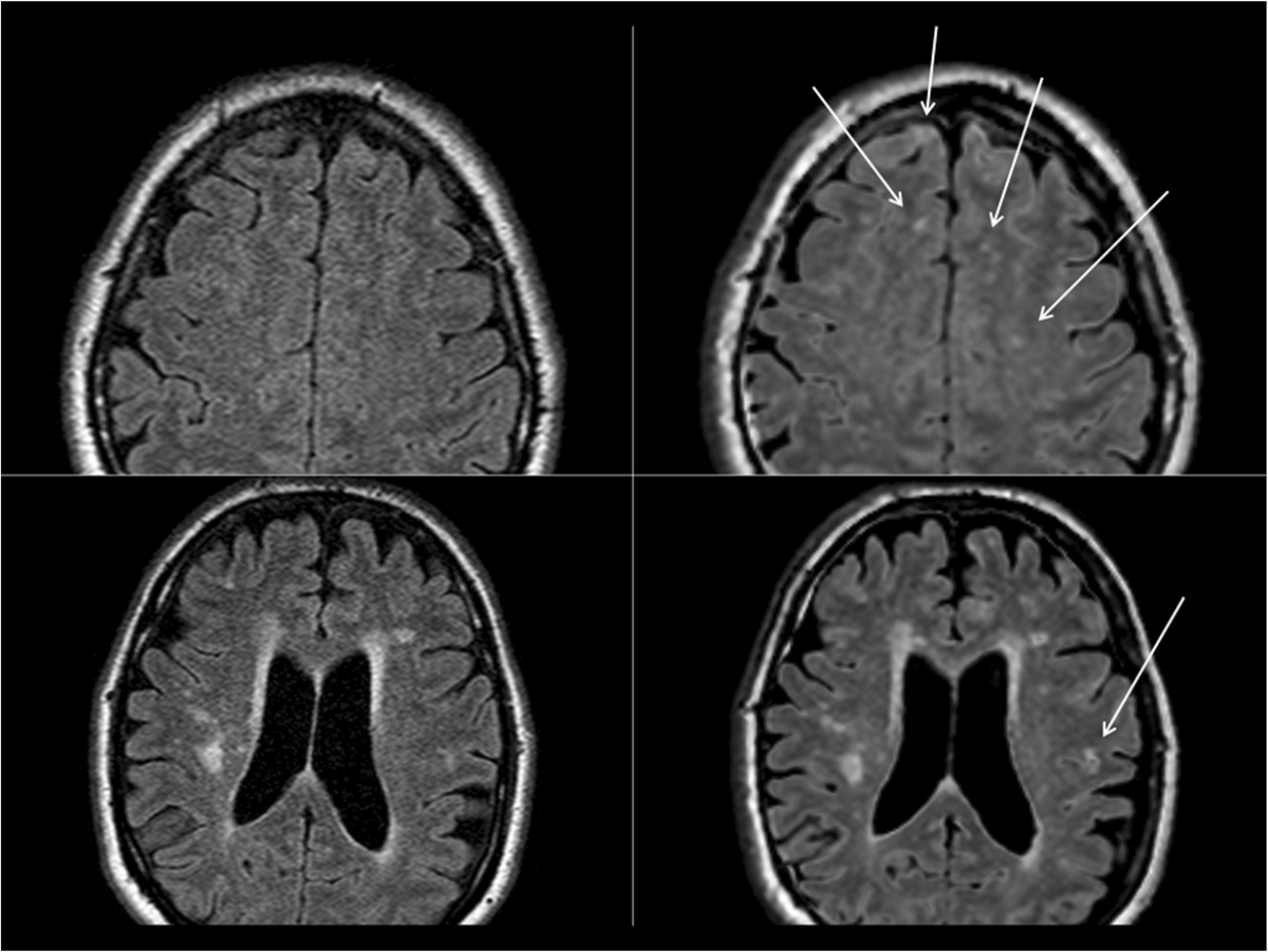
Conventional (*left column*) and synthetic (*right column*) FLAIR images. Suspicious MS lesions on synthetic images (*arrows*) are less obvious on conventional images
